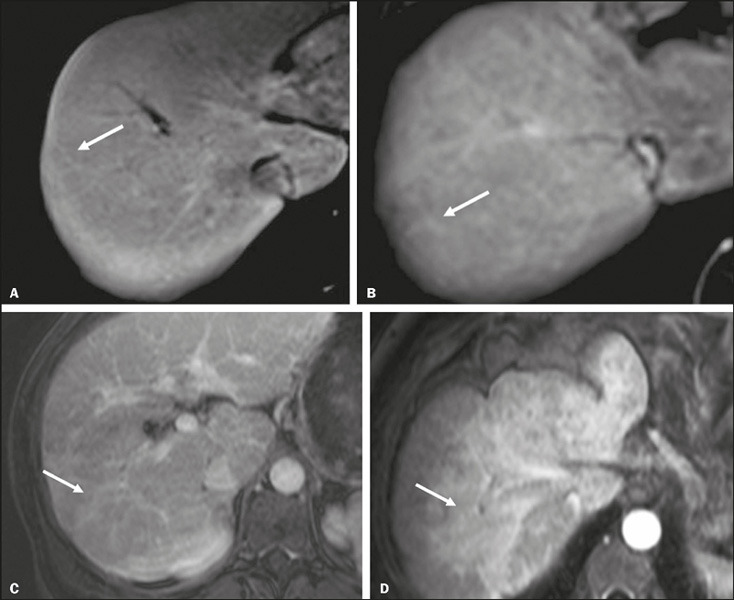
Grading of liver fibrosis by MRI in AIH. Fibrosis was characterized
